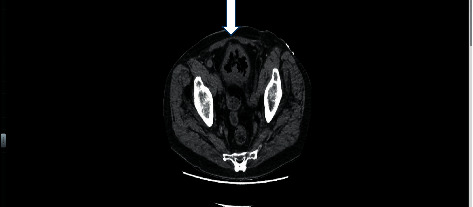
CT scan image showing a small perforation at the bladder dome and changes in keeping with emphysematous cystitis.
